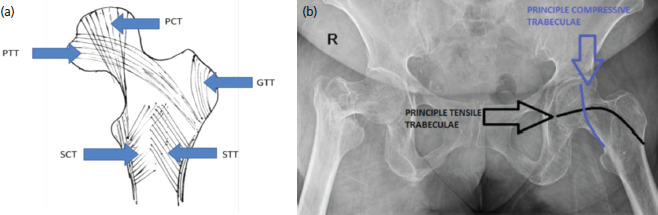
(a) Pictorial depiction of proximal femur trabecular pattern (b) Pelvis radiograph depicting the trabecular pattern of proximal femur.
